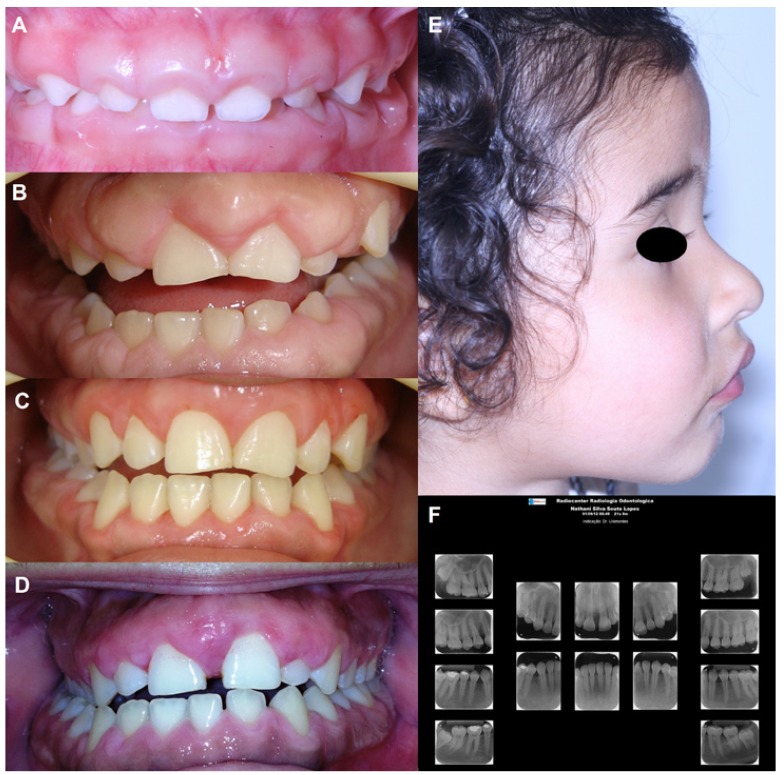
Clinical and radiographic characteristics of the family with Hereditary gingival fibromatosis. Gingival overgrowth in the anterior facial area of the proband (A) and her mother (B). In both, severe and generalized gingival enlargement involving both the maxillary and mandibular arches, covers almost two-thirds of the clinical crowns. The teeth are morphologically normal. (C) Aspect of gingiva after the last surgical intervention in the mother. (D). After seven years, the mother presents gingival overgrowth, indicating the recurrence of Hereditary gingival fibromatosis. (E) Proand had a marked cross bite with difficulties in the correct lip closure. (F) The panoramic radiography revealed any missing or malformed teeth of mother’s proband.
